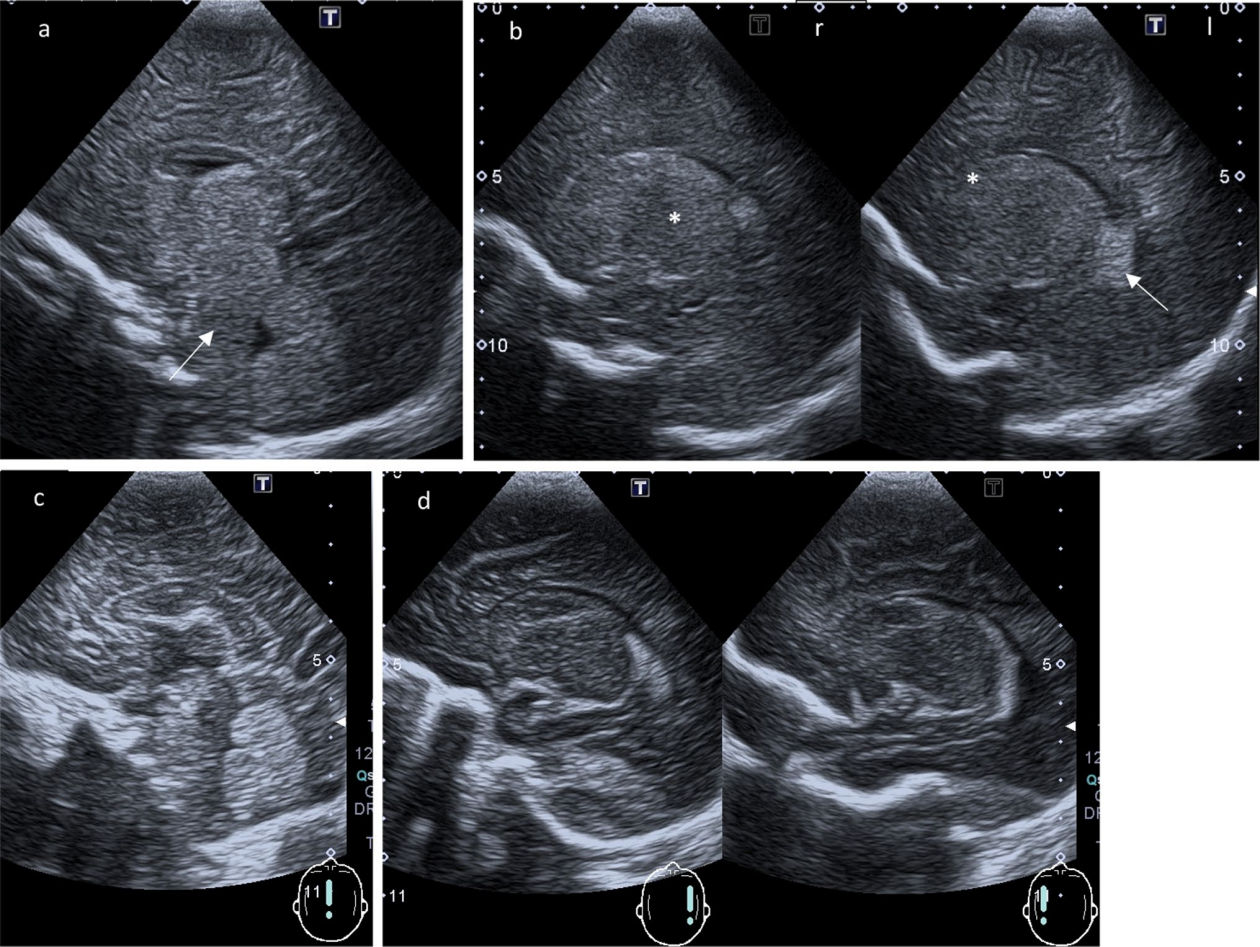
(Para-)sagittal ultrasound scans. a Midline sagittal plane: Increased echogenicity anterior to the fourth ventricle of pons and medulla (arrow). Third ventricle with edematous echogenic massa intermedia. b Parasagittal planes through the body of the lateral ventricles (right r and left l): Choroid plexus (arrow) remain highly echogenic in contrast to the normal echogenicity of thalamus (* b, r) and nucleus caudatus (* b, l). c, d Comparison to a normal age-matched child: Midline sagittal plane respectively parasagittal planes through the body of the lateral ventricles
